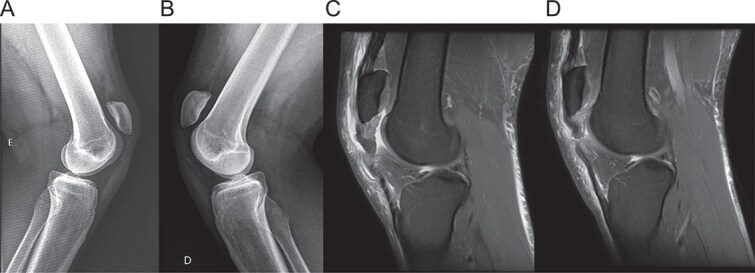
Preoperative imaging. (A, B) lateral radiographs of the right and left knee showing patella alta. (C, D) Sagittal T2-weighted MRI scans of the right and left knees, respectively, confirming complete proximal patellar tendon rupture with associated tendinosis.
